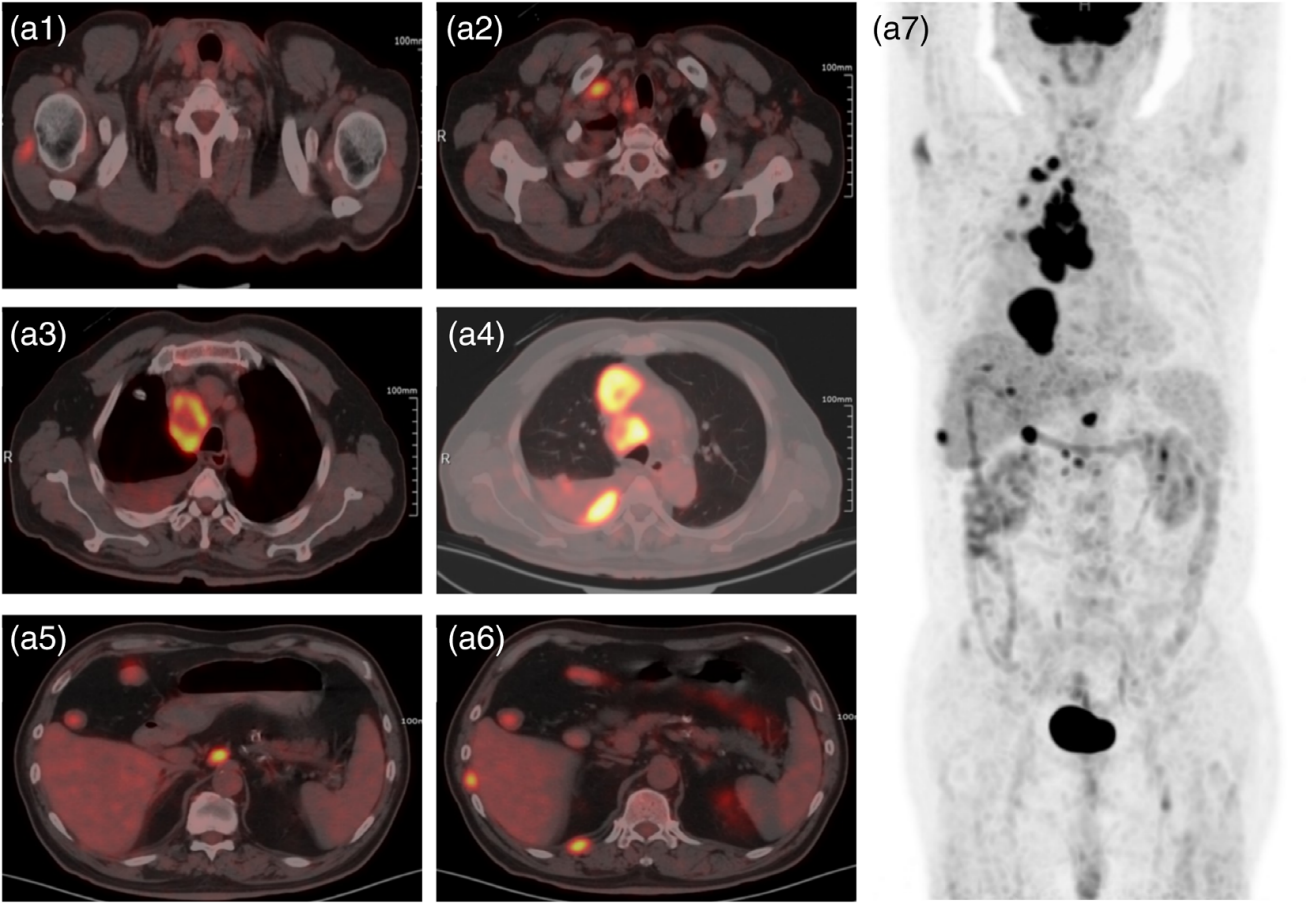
Pretreatment ^18^F‐fluorodeoxyglucose positron emission tomography/computed tomography (^18^F‐FDG PET/CT) images taken in May 2022. (a1) A slightly hypodense nodal shadow in the right shoulder deltoid, which does not exclude metastasis. (a2) Lymph nodes in the right clavicular area. (a3, a4) Intramediastinal and paratracheal lymph nodes with right pleural effusion. (a5) Parietal abdominal aorta and perivascular lymph nodes in the inferior abdominal aorta and inferior vena cava. (a6) Nodules under the right anterior wall and 11–12th intercostal space and multiple small nodular mass‐like thickening of the right pleura, considered to be pleural metastasis. (a7) The patient's whole body ^18^F‐FDG metabolic map.
